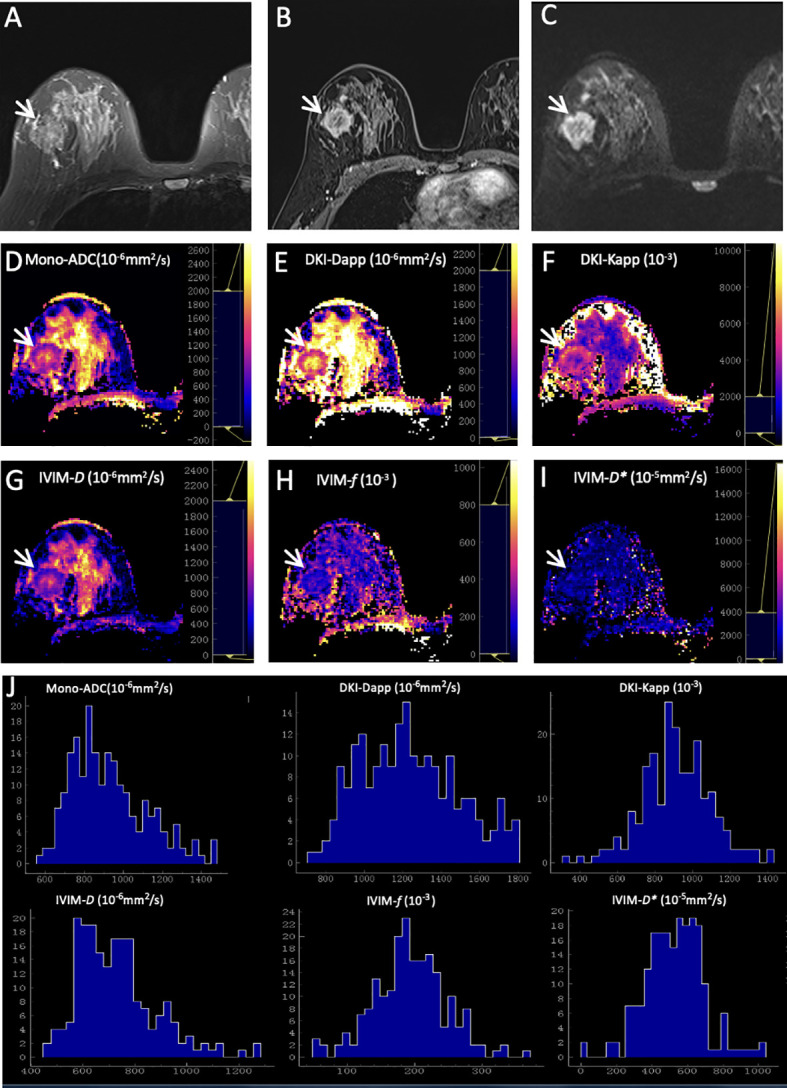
Representative images of a grade 3 invasive ductal carcinoma in the right breast of a 48-year-old woman. This tumor was with negative expression of estrogen receptor (0) and progesterone receptor (0), high Ki-67 (50%), and positive HER2 expression. (A) T2WI. (B) This mass is irregular and with obvious enhancement (arrow) on axial DCE-MRI. (C) This mass shows irregular shape, irregular margin, and heterogeneous signal on DWI (b-value = 1000 mm^2^/s) (arrow). ADC (D), Dapp (E), Kapp (F), *D*
